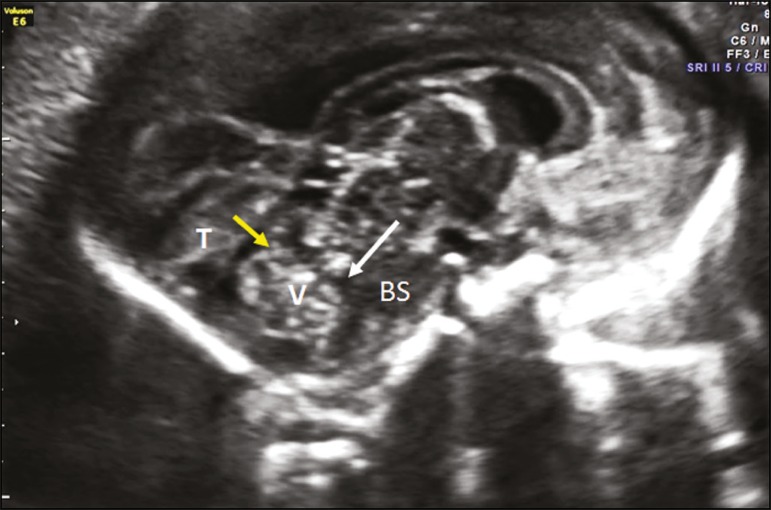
Ultrasound in the median sagittal plane, the best plane in which to assess the fetal posterior fossa (mainly for the differential diagnosis of cystic malformations). It is possible to identify the following: the brainstem (BS); the cerebellar vermis (V), with assessment of its morphology and biometry (craniocaudal and anteroposterior diameter); the primary fissure (yellow arrow); the fourth ventricle; the fastigium (white arrow); and the tentorium (T).
